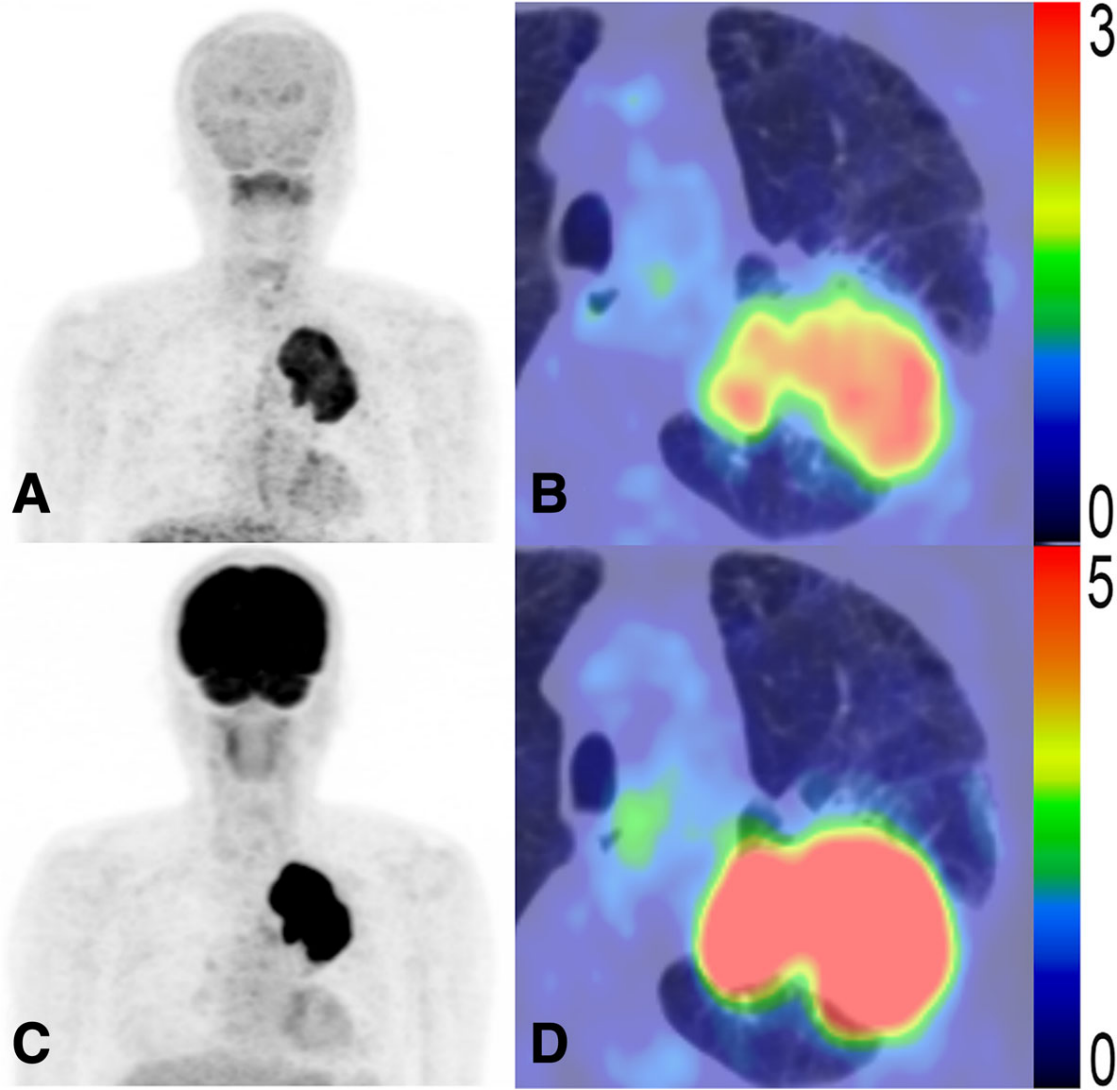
PET images of a 60-year-old male NSCLC patient at stage IIIB (T4N0M0) with high ^18^F-FAMT uptake (SUV_max_ = 3.6, MTV = 166.0 cm^3^, TLR = 315.4) (a, b) and high ^18^F-FDG uptake (SUV_max_ = 14.8, MTV = 209.0 cm^3^, TLG = 1442.1) (c, d). This patient was treated with chemotherapy and died 122 days later
